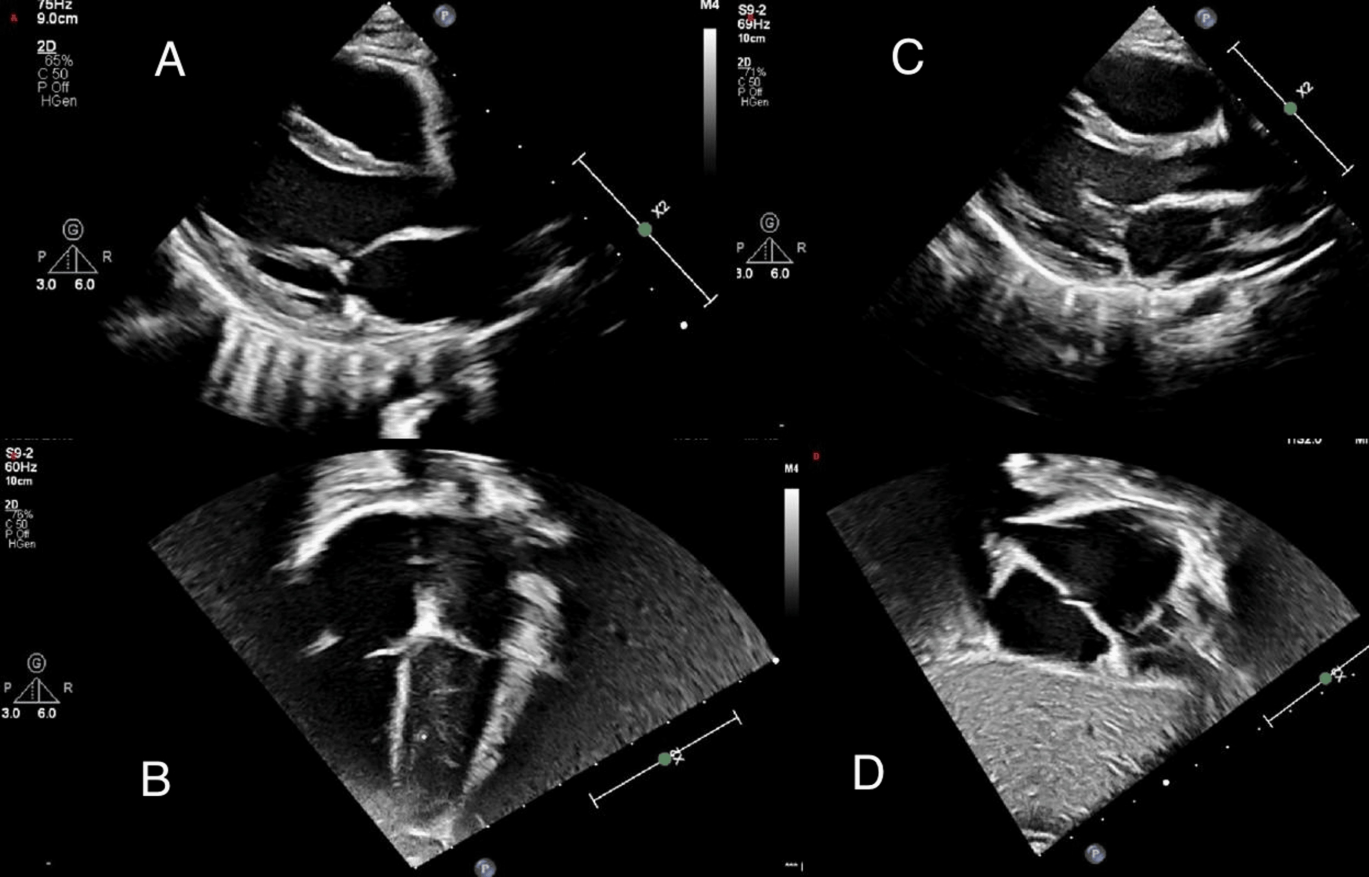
Post-Pericardiocentesis and Follow-Up Echocardiography Panels A, B: Post-pericardiocentesis parasternal long-axis and apical four-chamber views showing resolution of effusion. Panels C, D: Six-week follow-up parasternal long-axis and short-axis views demonstrating absence of residual effusion.
